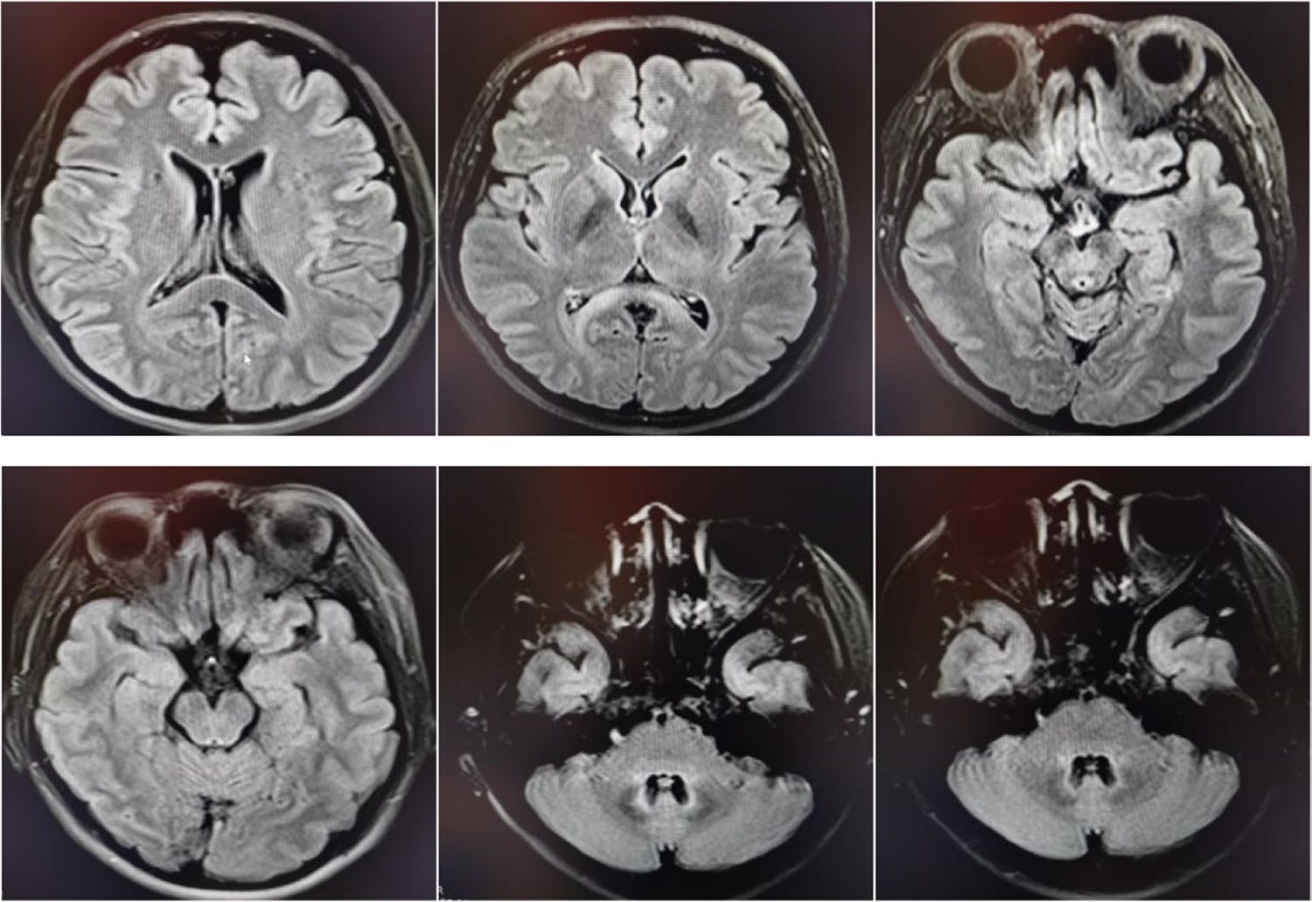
Multiple MRI lesions including enlarged left lateral ventricle and centrum semiovale lesions, symmetrical new lesions at the fourth ventricle, midbrain aqueduct, splenium of corpus callosum, and posterior horn of lateral ventricle
